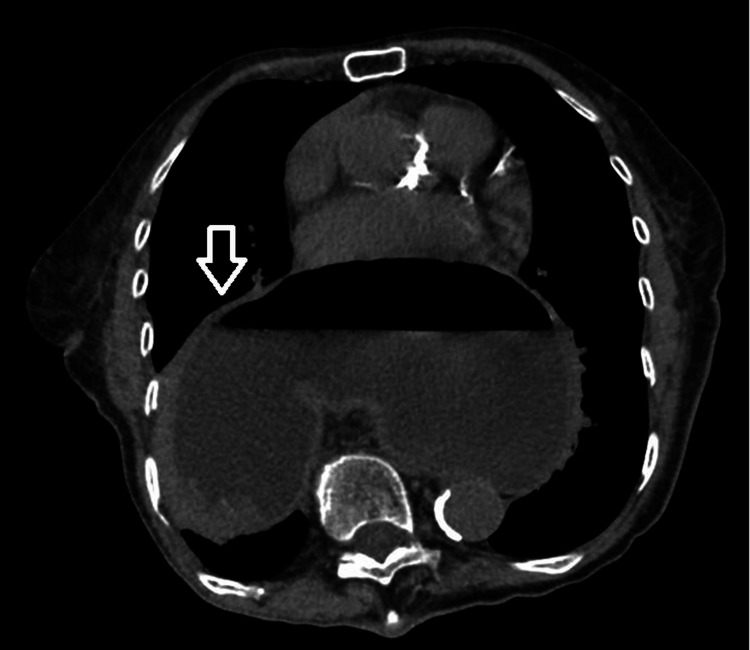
CT evidence of paraesophageal hernia with organoaxial gastric volvulus (arrow). Computed tomography (CT) of the abdomen and pelvis demonstrates a large hiatal (paraesophageal) hernia containing the stomach, with organoaxial gastric volvulus (the greater curvature positioned superior to the lesser curvature). The stomach is distended with an air-fluid level, raising concern for partial gastric outlet obstruction.
